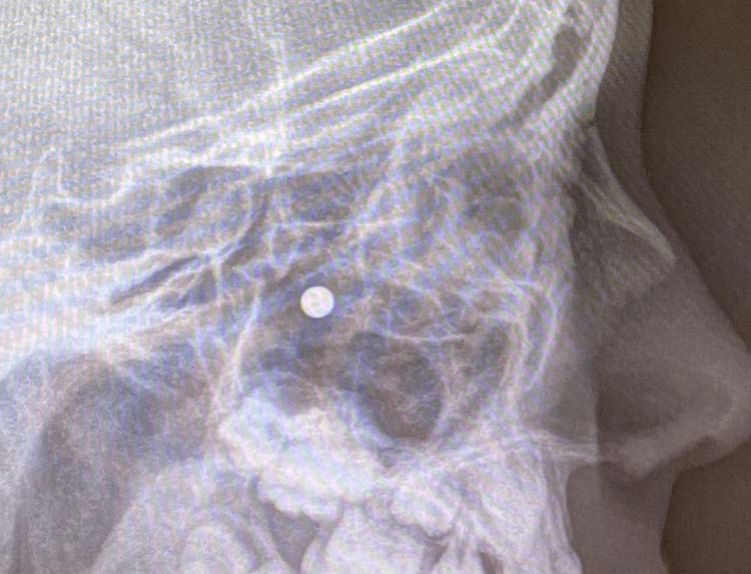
Sagittal view X-ray of sinuses.
